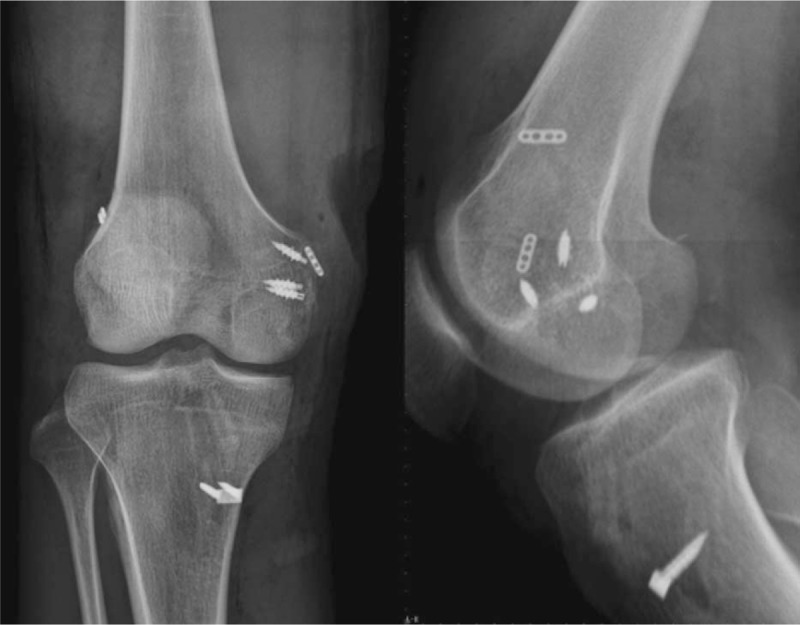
Anteroposterior (AP) and lateral radiographs immediately after surgery.
